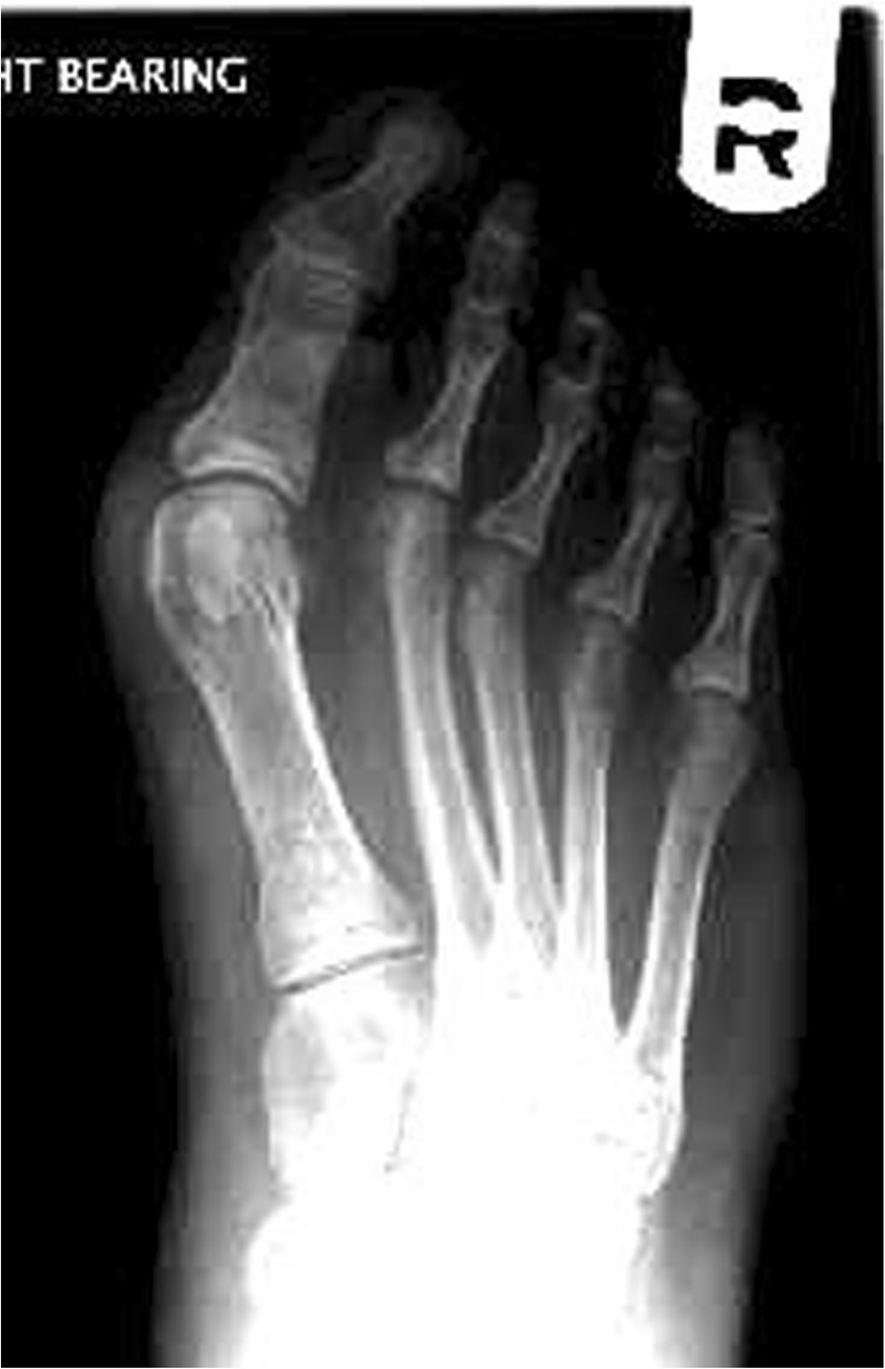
Survey case 1: Dorsoplantar weight-bearing radiograph of a patient’s right foot. History of complaints related to her hallux valgus deformity since one year, seeking surgical treatment after conservative measures had failed
